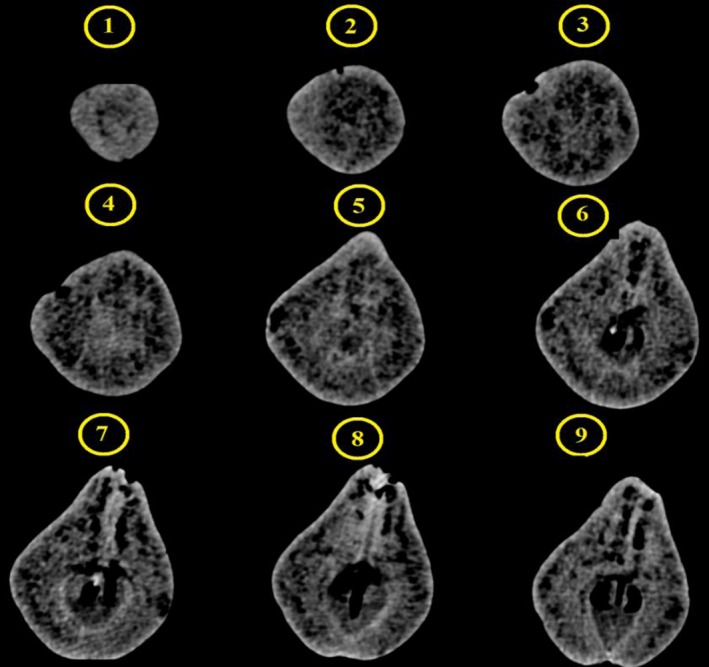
CT scan imaging process. There are 1–9 steps in creating the image, 1 first image and 9 final images
